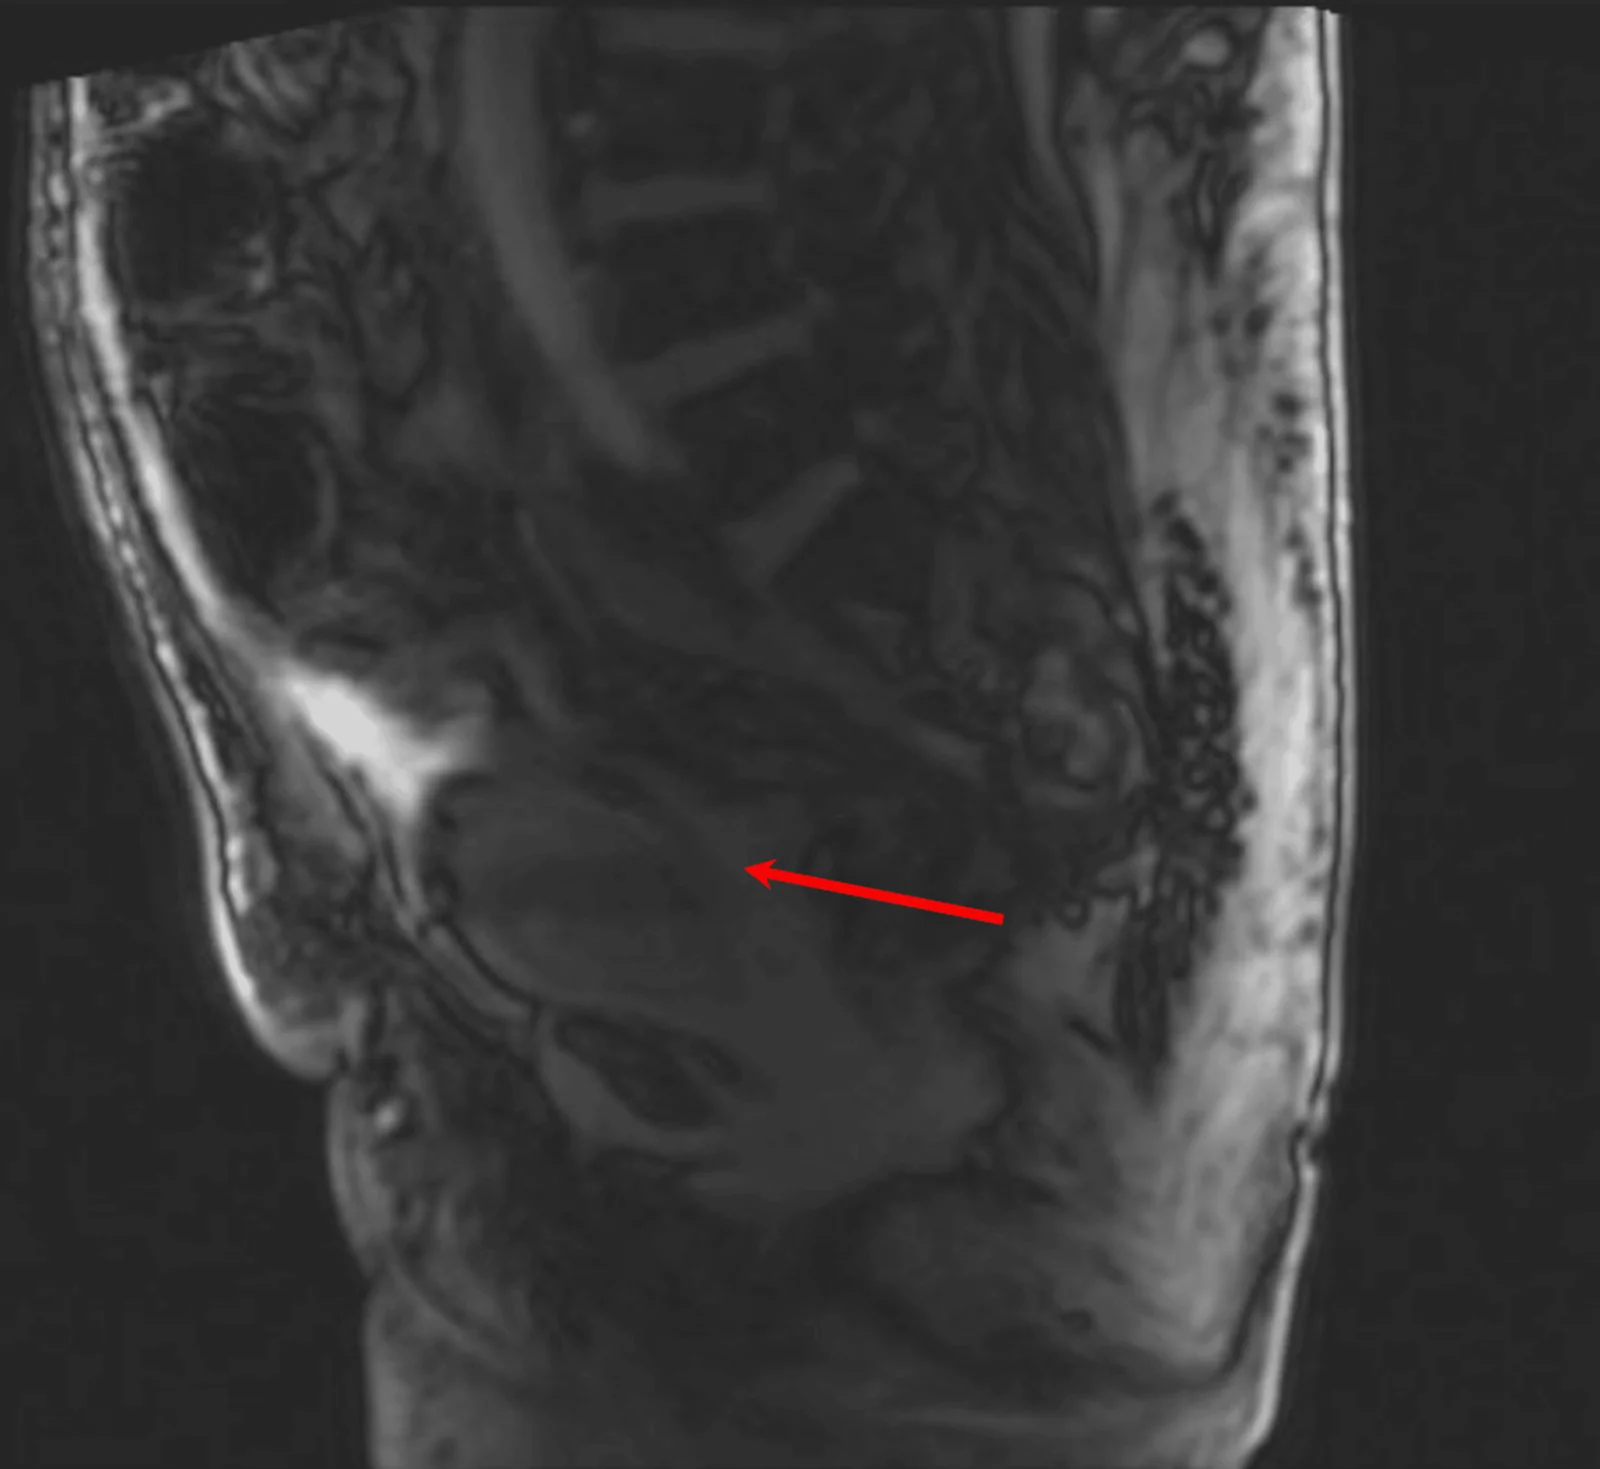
Axial CT of the abdomen and pelvis demonstrating a large pelvic mass Axial CT image showing a large heterogeneous pelvic mass (arrow), abdominal ascites, and anasarca. The organ of origin was indeterminate on CT. CT: computed tomography
